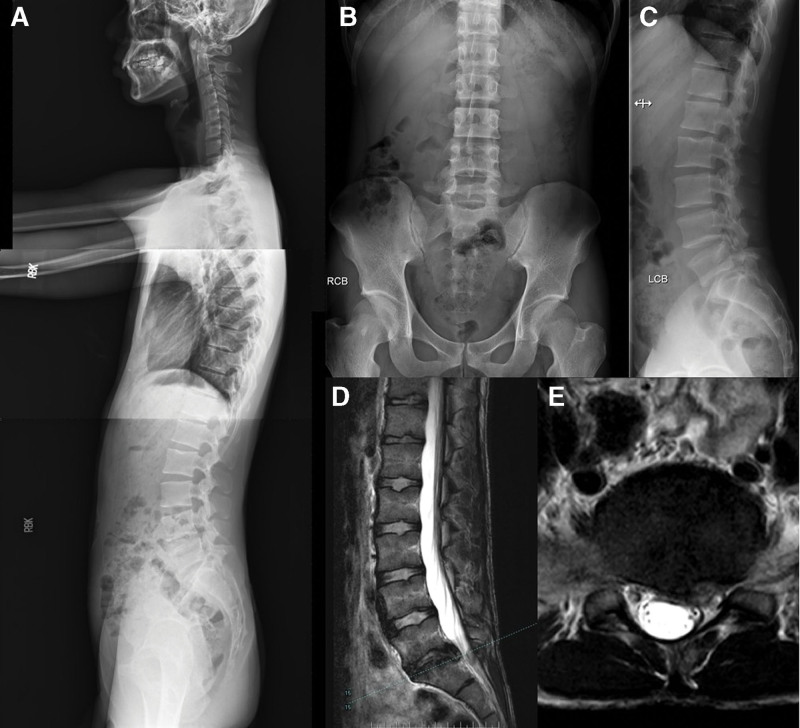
A 23-years-old male presented with low back pain and a 2-year history of painful numbness radiating to the left lower limb. (a, b, c) Radiographs of the whole spine showed the presence of the sixth lumbar vertebra. (d, e) Preoperative MRI showed L6-S1 disc herniation to the left side. MRI = magnetic resonance imaging.
